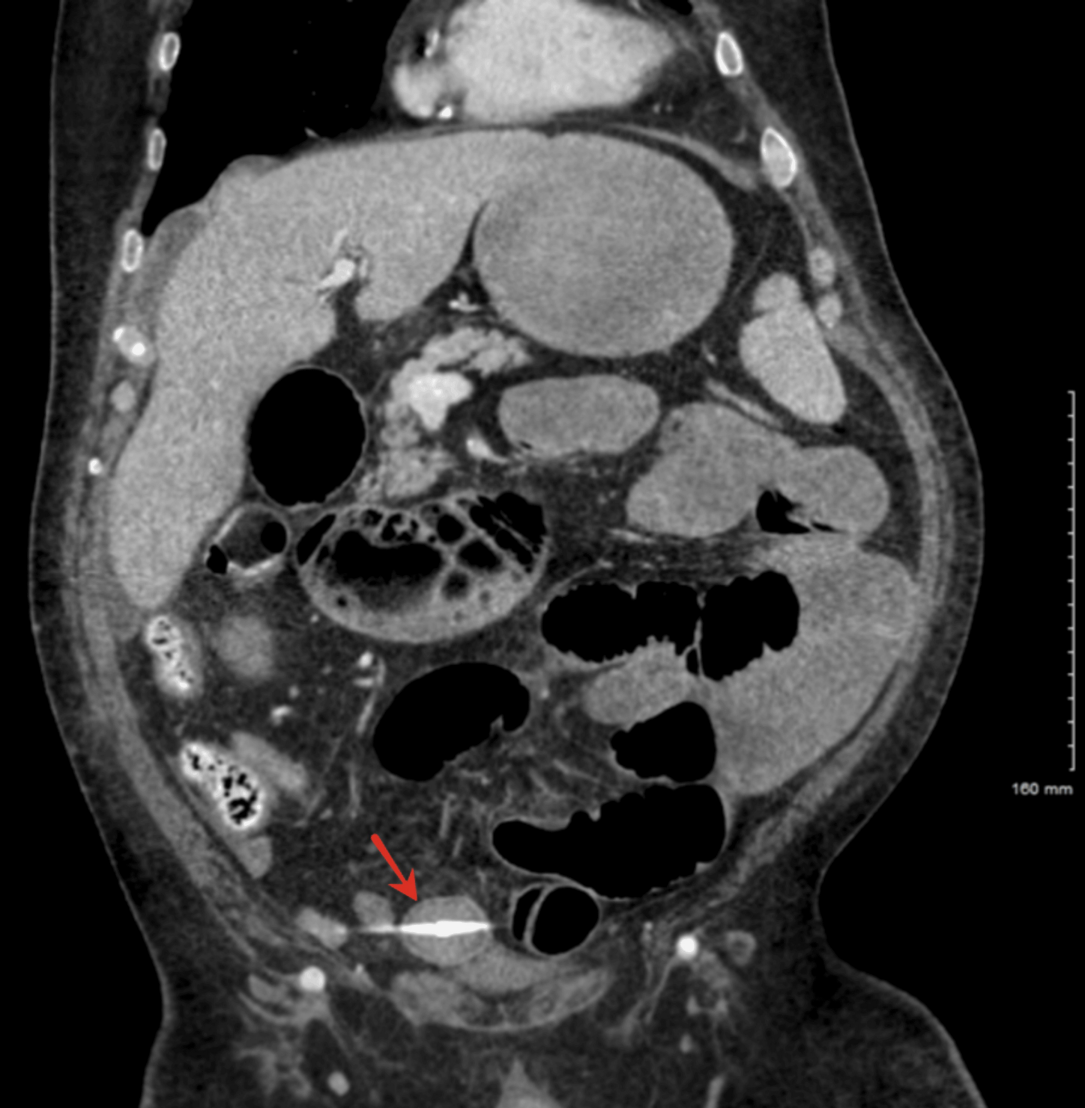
Coronal CT image of the abdomen and pelvis with oral contrast showing the obstructing gallstone within the distal ileum, consistent with gallstone ileus
